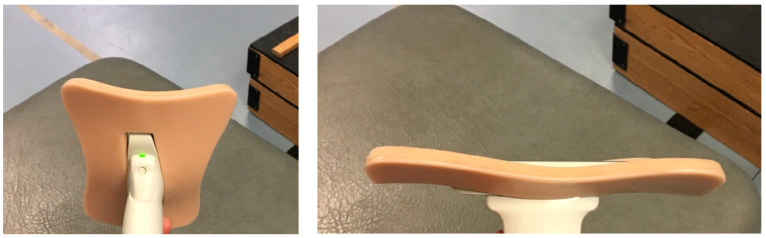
Custom-designed cast, housing a 10 cm ultrasound probe.
